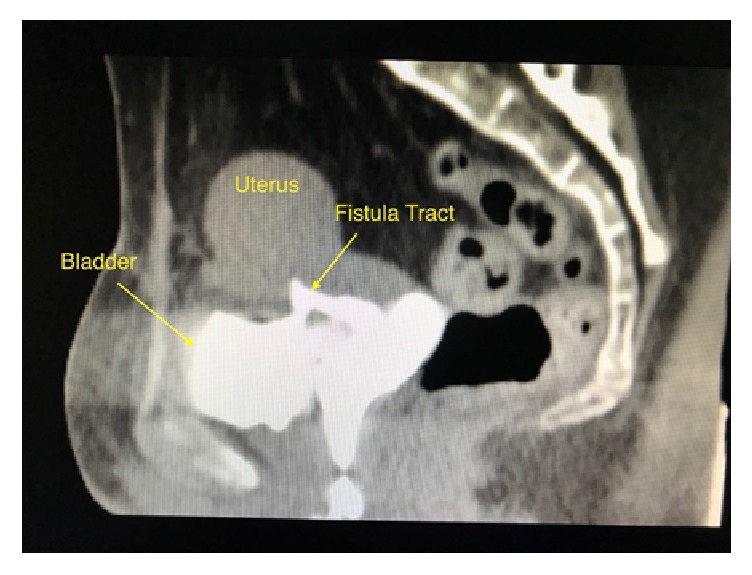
Sagittal view of contrast-enhanced CT scan demonstrating the connection between bladder and uterus.
